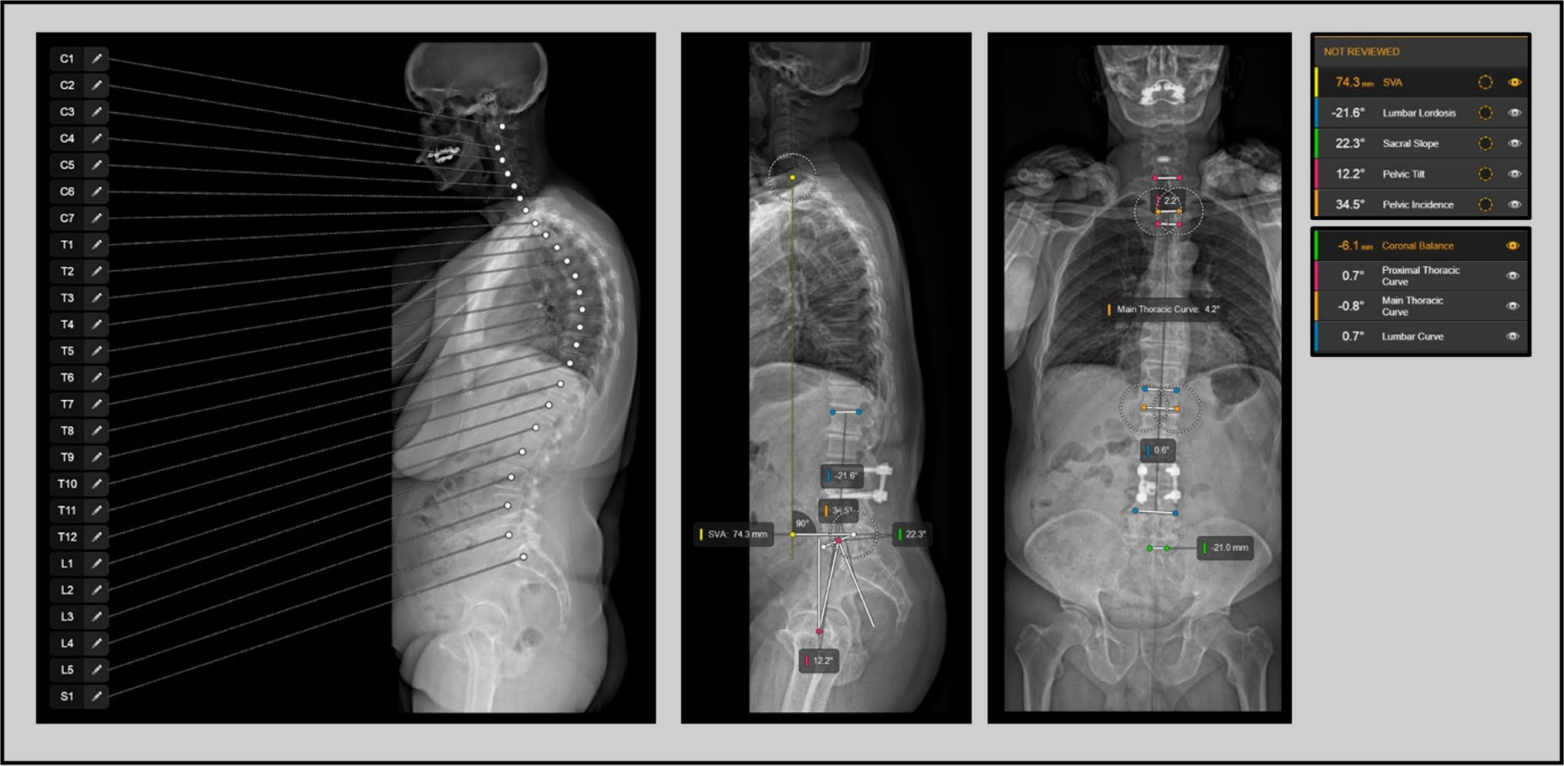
Representative scaled sagittal and coronal radiographs showing the vertebral bodies as identified by the software and the spinopelvic parameters derived
